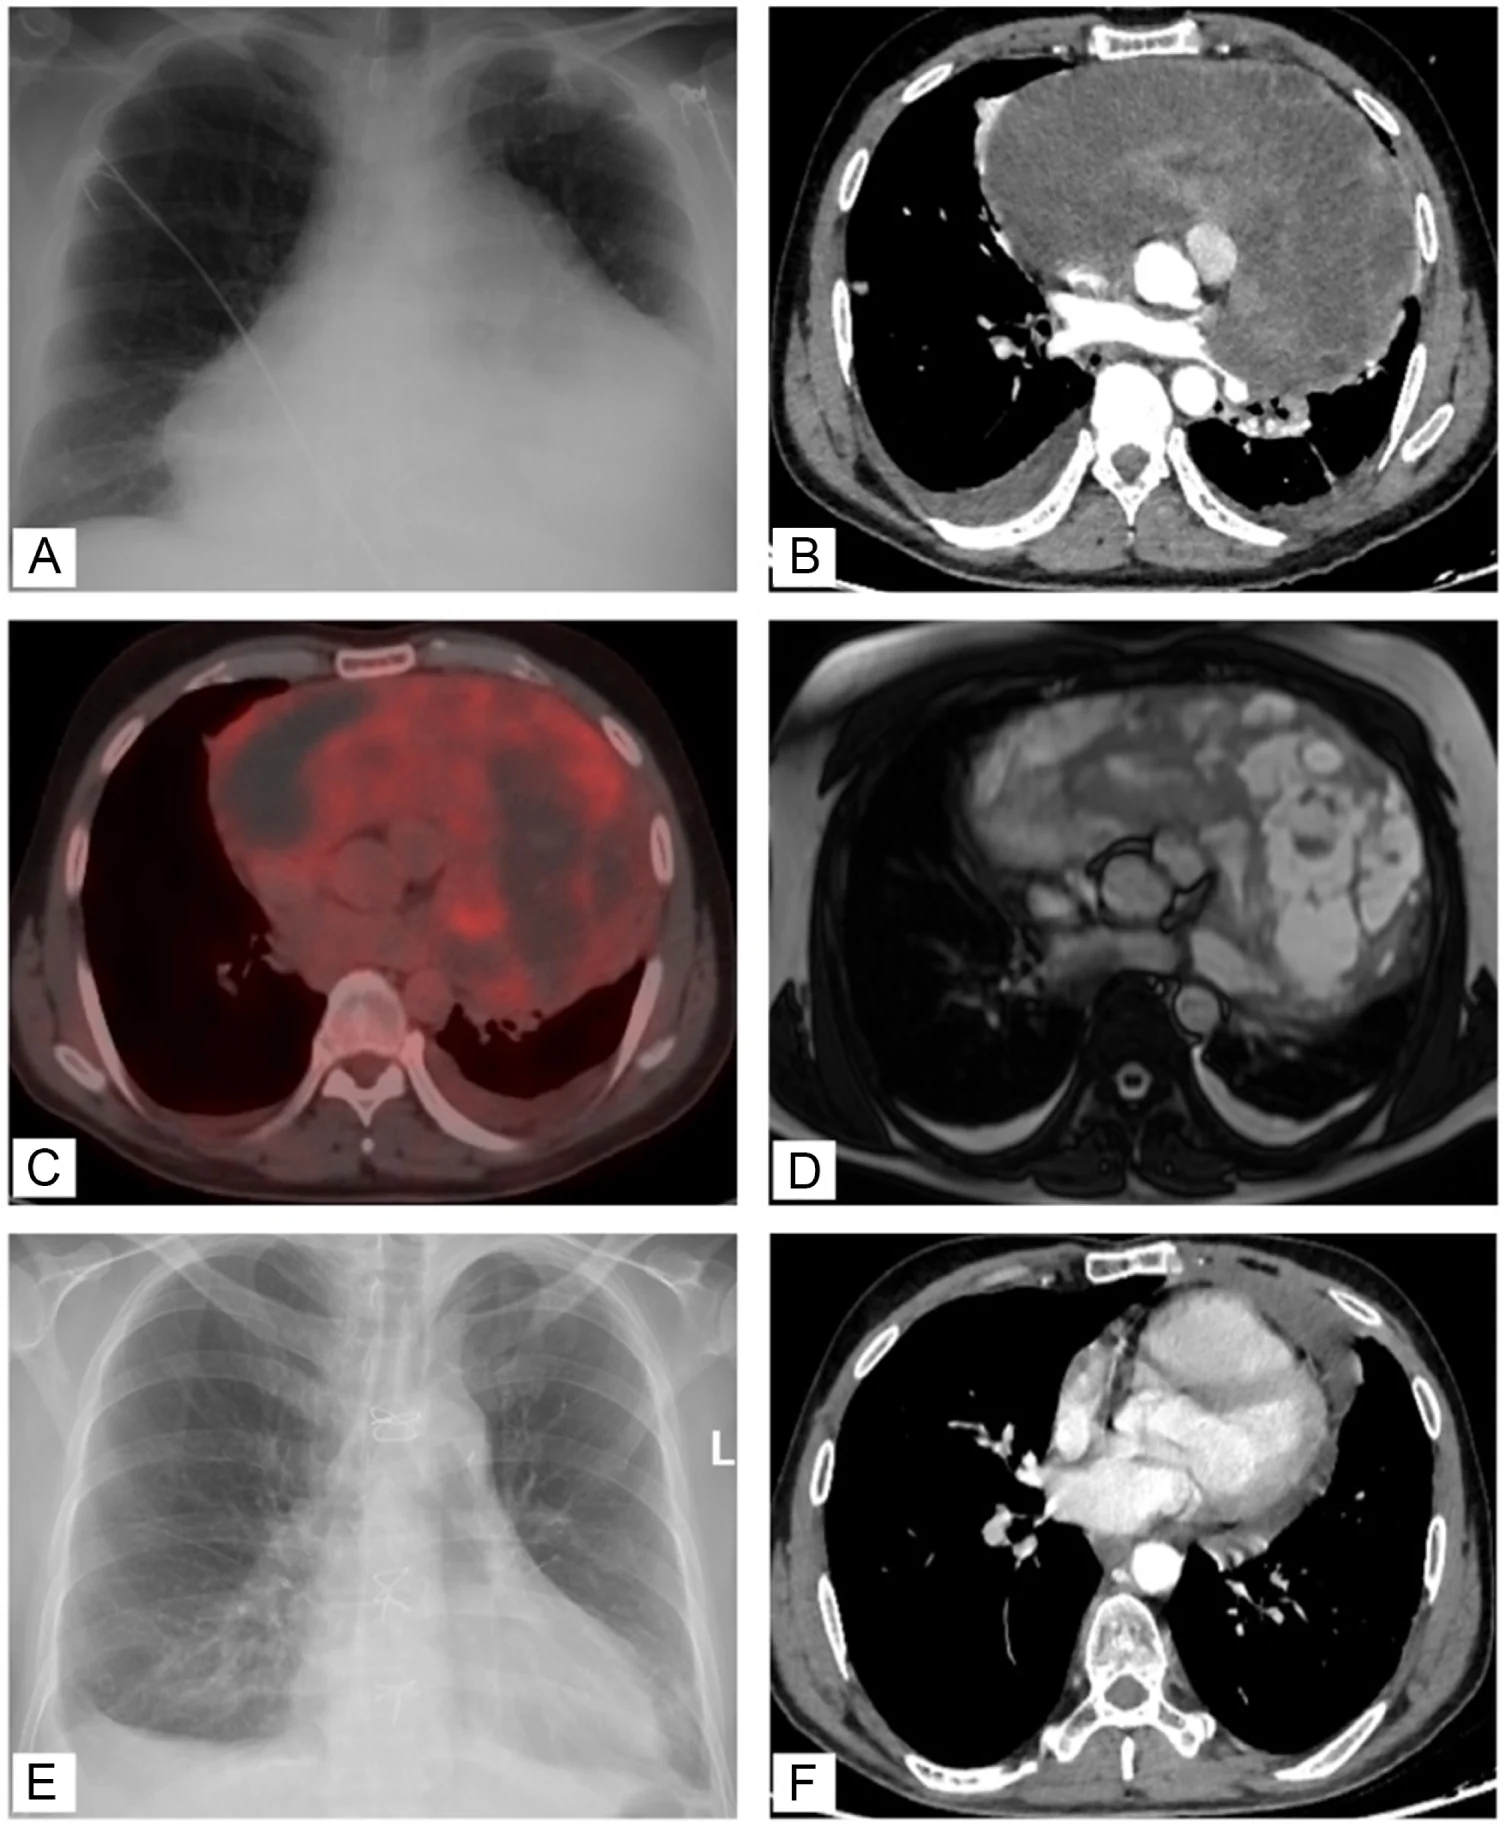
(A) Preoperative chest X-ray, (B) preoperative thorax CT, (C) preoperative PET/CT, (D) preoperative thorax MRI, (E) postoperative chest X-ray, (F) postoperative thorax CT. PET-CT, positron emission tomography-computed tomography; MRI, magnetic resonance imaging.
